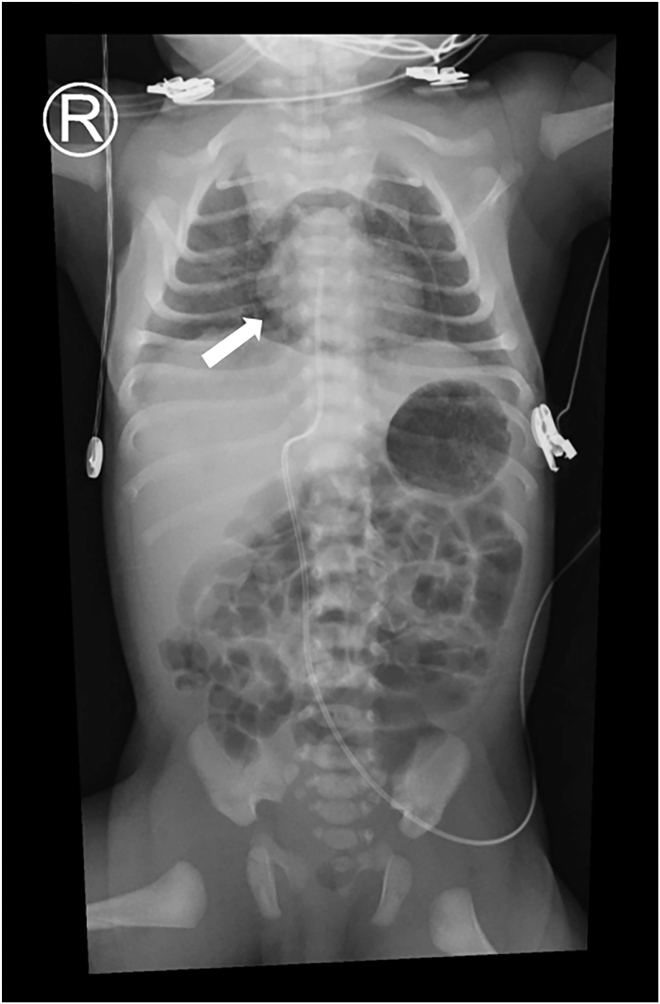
Pericardiocentesis was deferred. Clinical improvement enabled extubation at 10 hours. Serial radiographs showed complete spontaneous resolution of the pneumopericardium and concurrent pneumonitic changes by 40 hours (Figure 2). The infant recovered uneventfully and was discharged on day 7 and remained well with normal growth and development at the 1-month follow-up.
